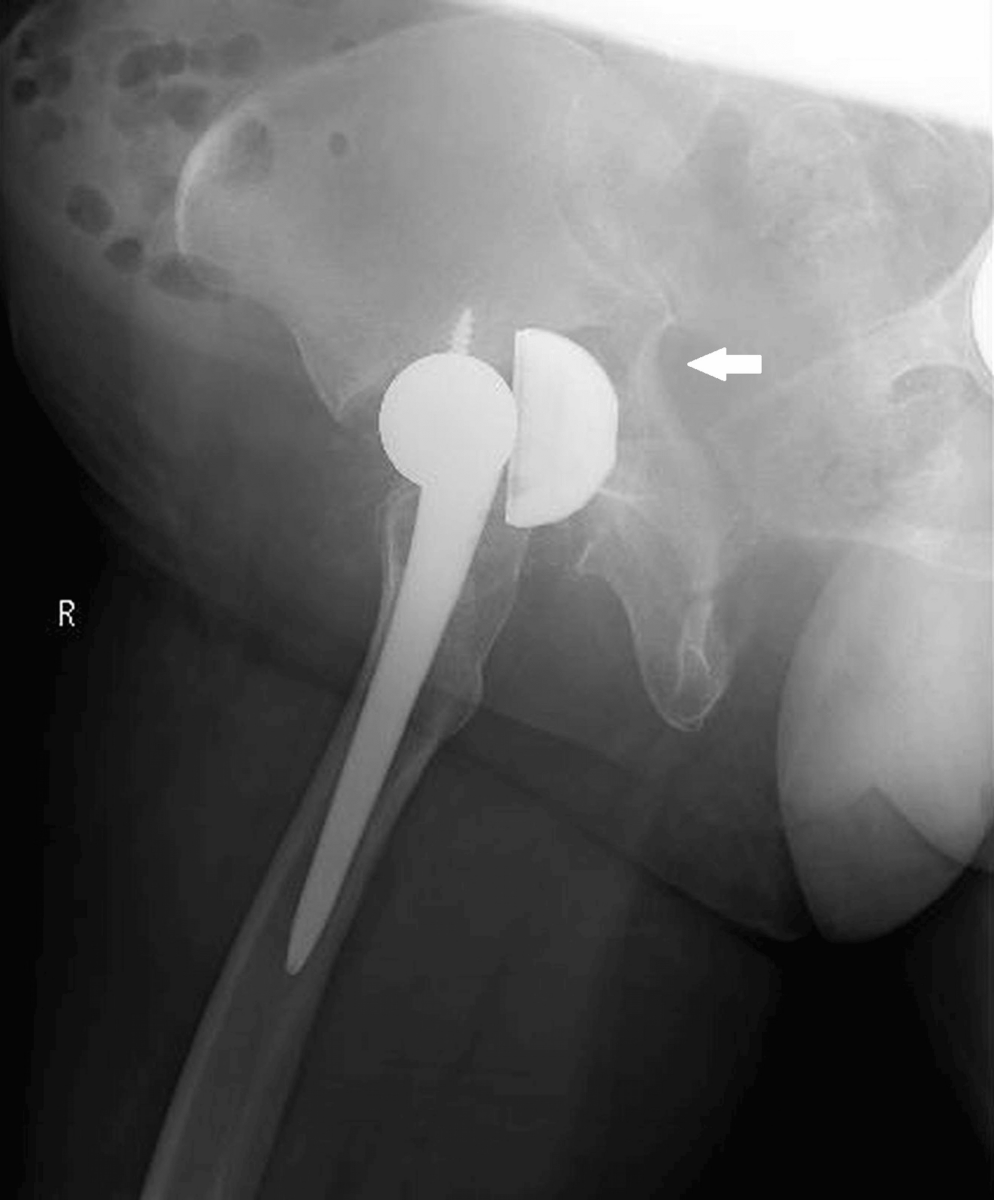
Plain radiograph of the pelvis after the secondary fall (lateral view). The fracture displacement had progressed (arrow), and the acetabular cup had become dislodged.
